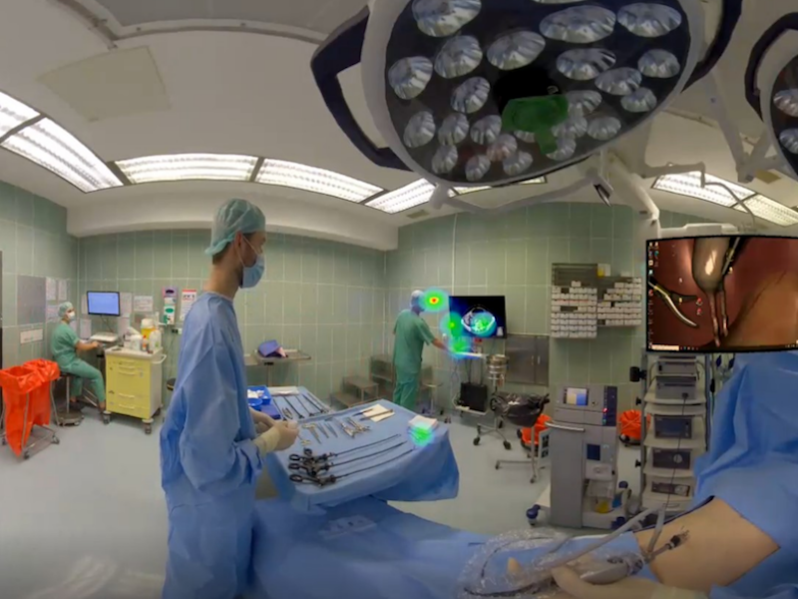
Part of the participant’s view in immersive virtual reality is shown. The scrub nurse is standing beside the participant. On the left edge of the picture, the laparoscopy monitor with an exercise is shown. In the background, a colleague is demonstrating a CT scan of the next emergency patient with a bowel obstruction (audio-visual distraction). CT: computed tomography.
